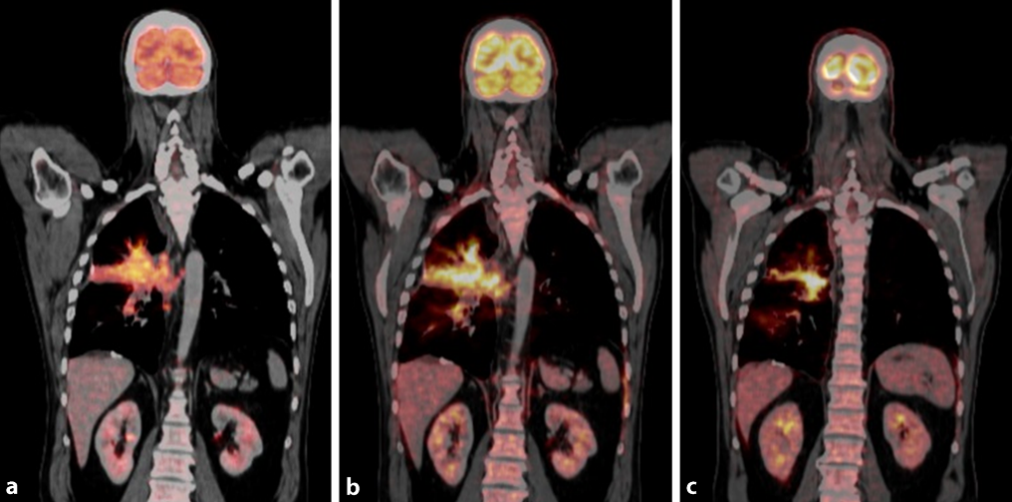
Pseudoprogression in 54-year-old man with non-small cell lung cancer receiving immune checkpoint inhibitor therapy. a Coronary fluorodeoxyglucose positron emission tomopraphy/computed tomography (^18^F-FDG-PET/CT) imaging obtained before therapy demonstrate ^18^F-FDG avid malignant tumor in the right lung. b 5 weeks after treatment initiation tumor size and FDG uptake increased. Therapy was continued and 6 weeks thereafter tumor size shrinkage and a reduced ^18^F-FDG uptake were observed (c)
